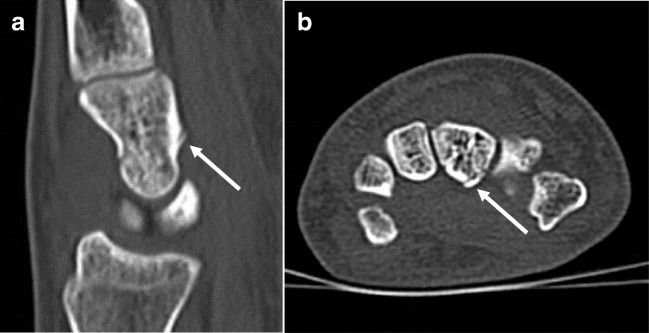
False-positive CT of the wrist of a 32-year-old woman who fell on her left arm in dorsiflexion. At physical examination, she had excoriations and pressure pain at the level of the dorsal radius. There was no fracture at CR. Although at CT there was suspicion of a fracture of the capitatum (a and b,* arrow*), the patient was treated with a pressure dressing because she refused cast treatment. At follow-up, pain decreased within 1 day. In retrospect, the finding at CT was caused by a vascular channel, without any visible swelling or induration in the surrounding soft tissue
